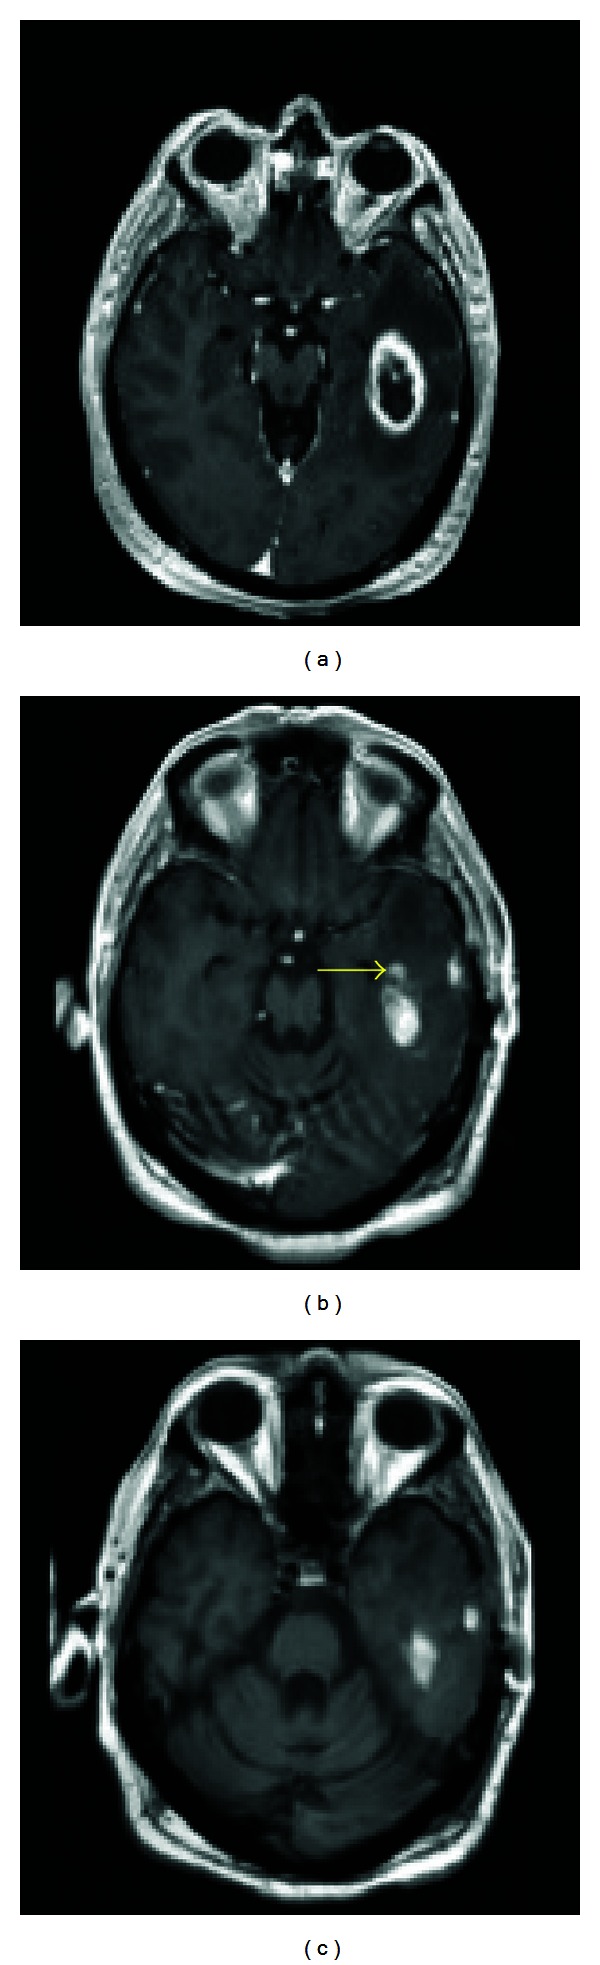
Preoperative and postoperative images. (a) Preresection T1 axial MRI with contrast showing a temporal lesion with an estimated 5.0 cubic centimeter volume. (b) Immediate postresection T1 axial MRI with contrast showing a slight amount of residual enhancement at the anteromedial margin of the resection cavity. (Yellow arrow indicates residual tumor area not present in (c)). (c) The corresponding noncontrast enhanced T1 MRI image taken at the same time demonstrates that the posteromedial and lateral hyperintensities are in fact blood products and postoperative change rather than gross residual tumor. A 93.3% volumetric resection was achieved.
